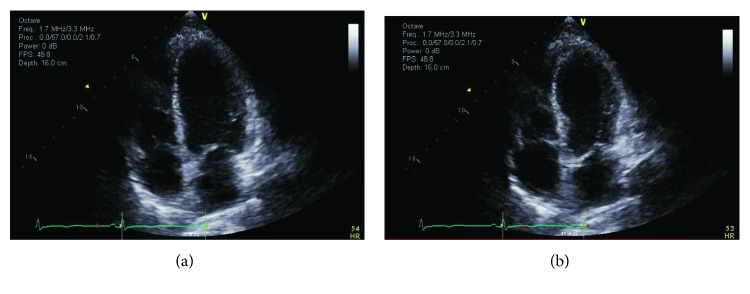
Echocardiographic images demonstrating end-diastole (a) and end-systole (b). Basal and apical thickening with sparing of the midchamber segments indicates a midventricular variant of TTS.
